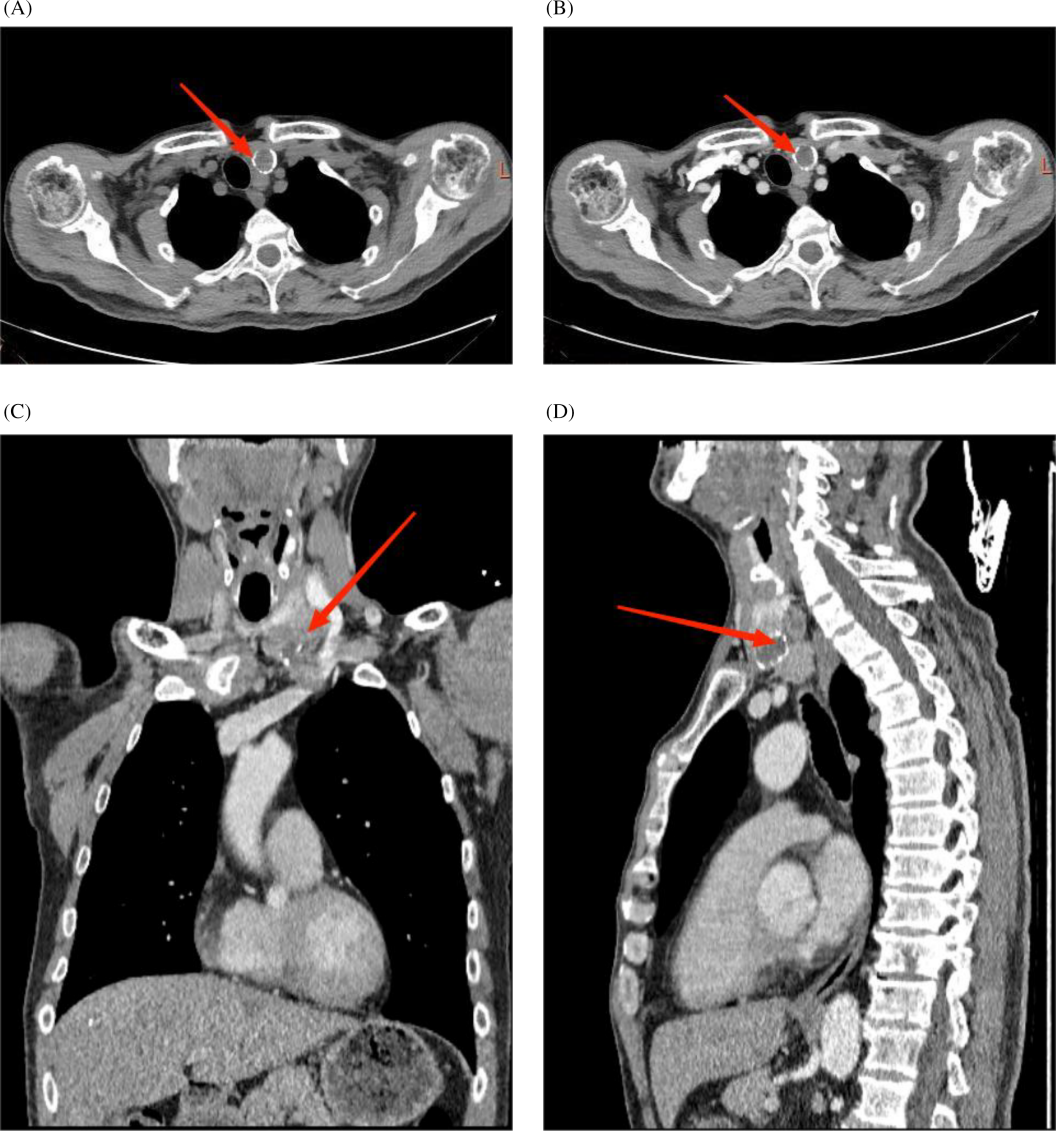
Neck computed tomography (CT) and contrast enhanced computed tomography (CECT) scan: (A) CT shows an enlarged left thyroid lobe within an irregular slightly hypointense shadow and circular calcification foci and a slightly rounded hypointense shadow posterior to the left inferior thyroid lobe; (B) CECT shows enhanced scanning of low‐density shadows of the left thyroid lobe; (C) coronal view of the thyroid shows an irregularly shaped left cervical mass protruding downward into the left paratracheal space and compressing the jugular vein; (D) sagittal view of the left thyroid lobe shows the papillary mass growing backward into the anterior esophageal space.
